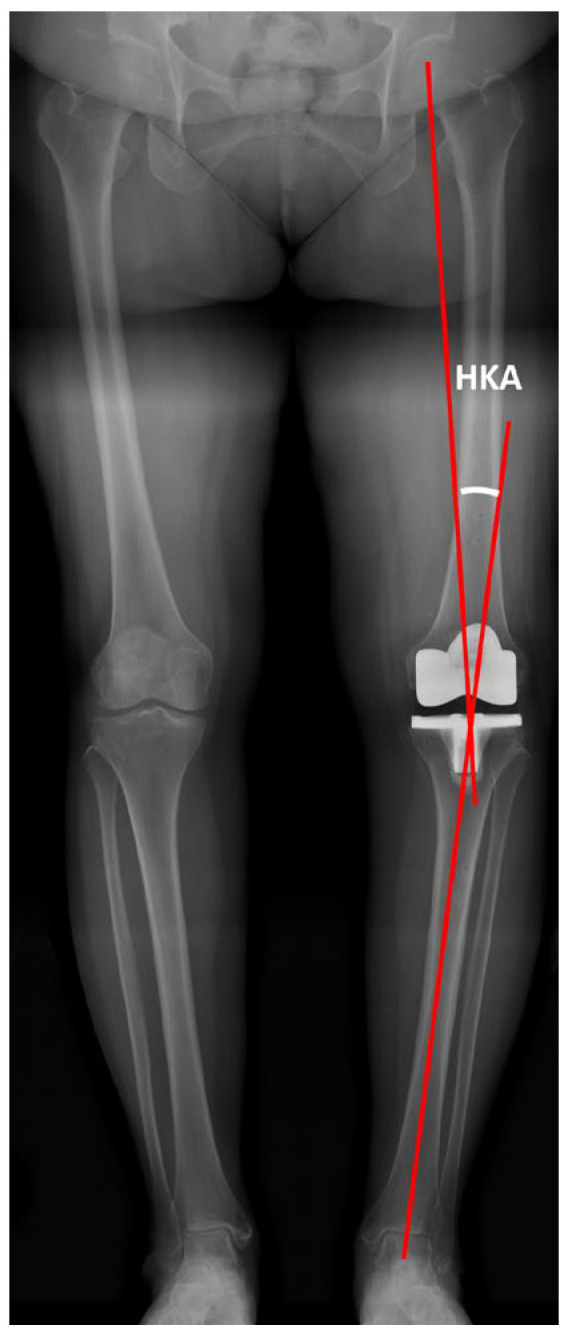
Measurement of the hip–knee–ankle (HKA) angle on full-length standing anteroposterior radiograph.
